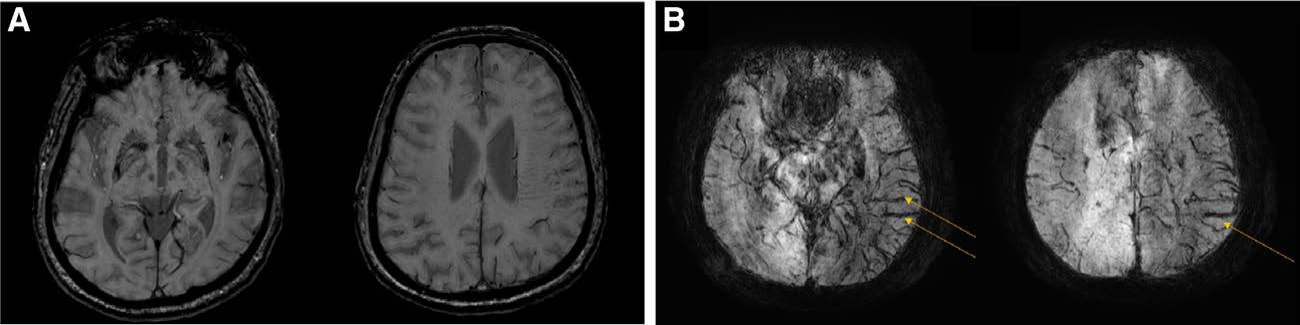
Examples of collateral flow evaluation with susceptibility-weighted imaging (SWI), according to the study of Lee et al (A) SWI of the patient as in Figure 2A, and it was judged to be a good collateral channel consistent with computed tomography angiography (CTA). (B) SWI images of the patient as in Figure 2B, and it was judged to be a poor collateral channel, which is the same as in CTA. In this manuscript, 2 cases were shown to present a representative image, so the collateral channel judged by CTA and SWI was the same. However, in some cases, the collateral channel judged by CTA and SWI did not match.
